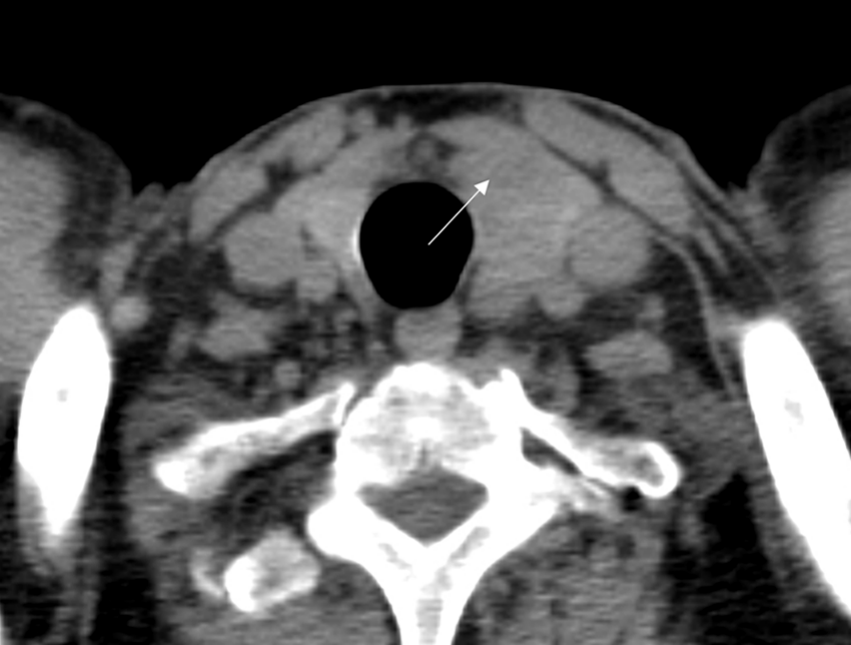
CT images of adult-type rhabdomyoma of the thyroid. Plain CT images revealed the left thyroid lobe was obviously enlarged, and there was a lesion (arrow) with slightly low density in the left thyroid lobe.
